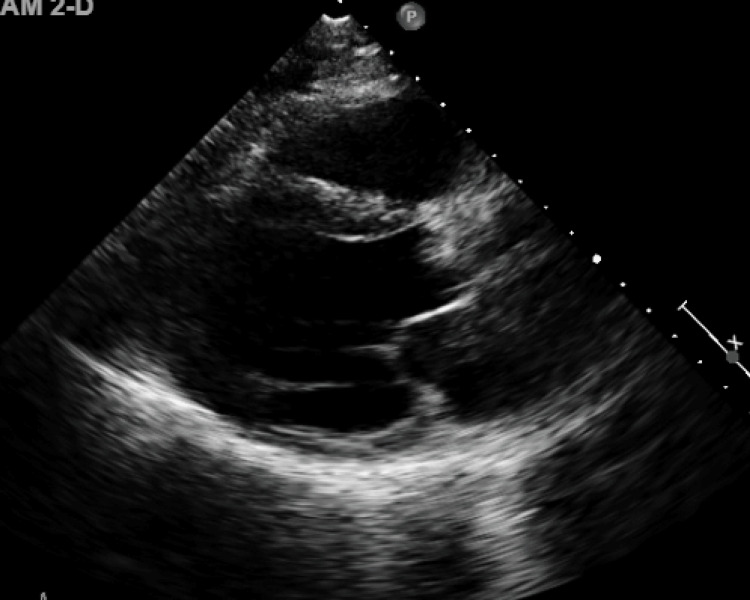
Repeat 2D ECHO revealed LVEF of 40-45% and severe aortic stenosis 2D ECHO: two-dimensional transthoracic echocardiograph; LVEF: left ventricular ejection fraction
